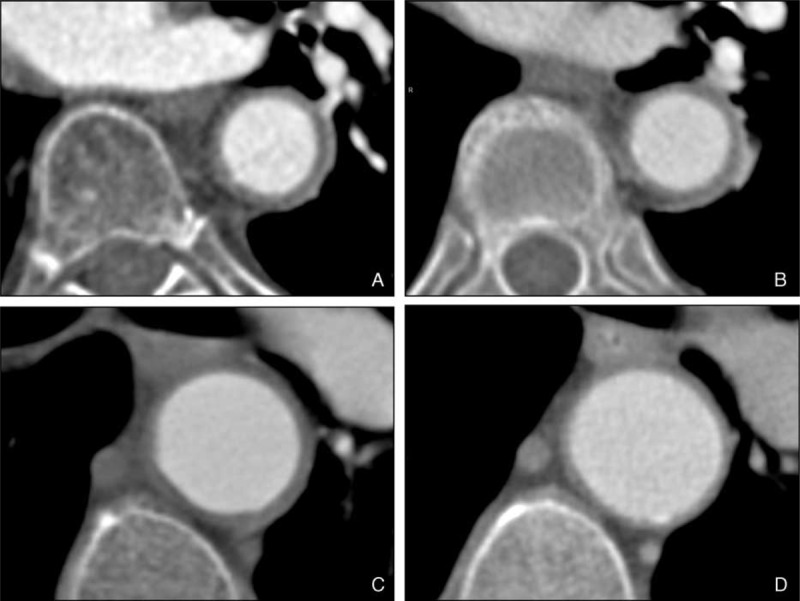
CTA of a newly diagnosed patient with GCA showing significant aortic wall thickening (panel A) with contrast enhancement in the late venous phase, sparing the intimal layer (panel B). Persistent aortic wall thickening after 1-year of treatment, devoid of contrast enhancement in the late venous phase (panels C and D).
